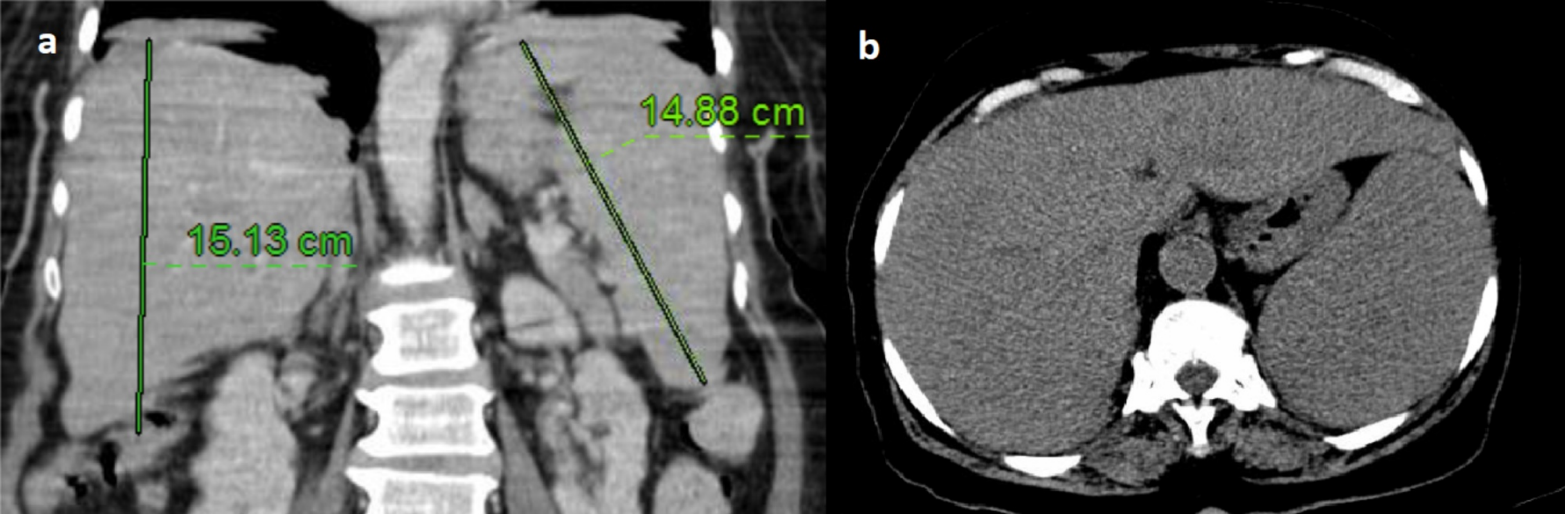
Computed tomography (CT) abdomen with contrast. (a) Coronal section showing hepatosplenomegaly. (b) Axial section showing no discrete masses.
